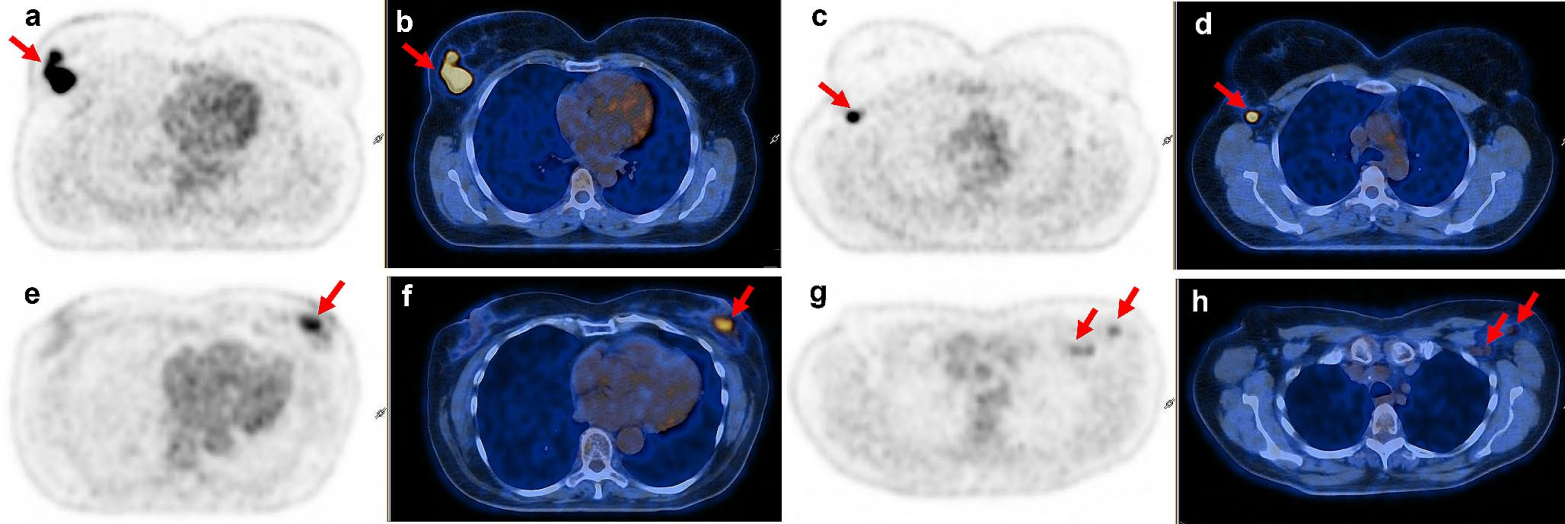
Pretreatment [^18^F]FDG PET only and fusion PET/CT images of two patients with HER2-negative BC and ipsilateral ALN involvement. a, b, c, d: TN BC with pCR after NAC. a, b: Primary tumor (SUV_mean_=8.21; CoV = 0.54); c, d: ALN (SUV_mean_=3.30); e, f, g, h: Luminal-B like BC with non-pCR after NAC; e, f: Primary tumor (SUV_mean_=3.32; CoV = 0.17); g, h: ALN (SUV_mean_=2.22)
